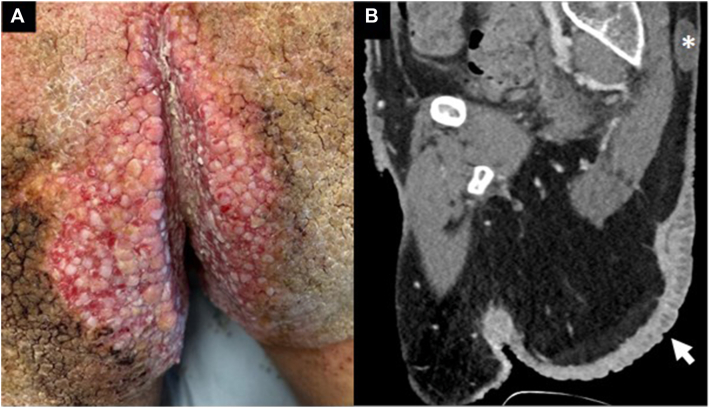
A, Erythematous, hyperkerotic plaques with erosion and purulent drainage involving the gluteal cleft consistent with secondary infection. B, Computed tomography of the pelvis demonstrating a gluteal abscess (∗) and thickened hyperkeratotic skin consistent with Darier disease (*arrow*).
